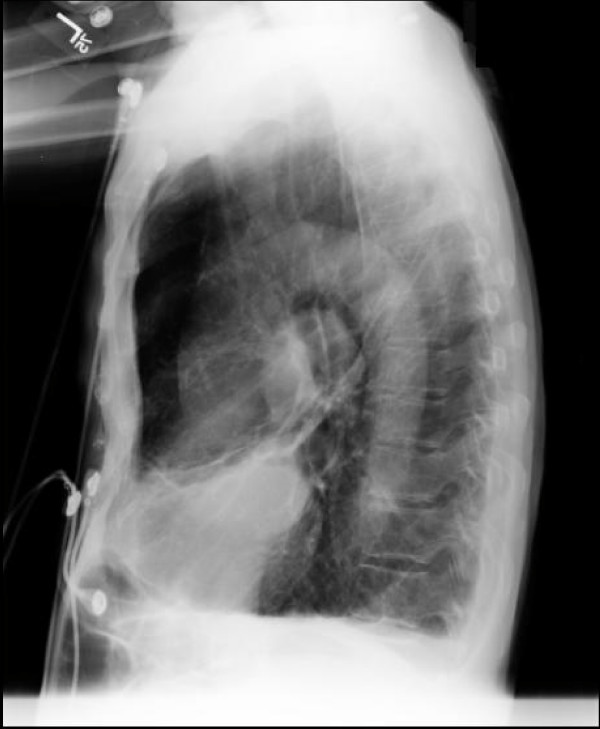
Chest radiograph displaying extensive calcification of the aorta.
